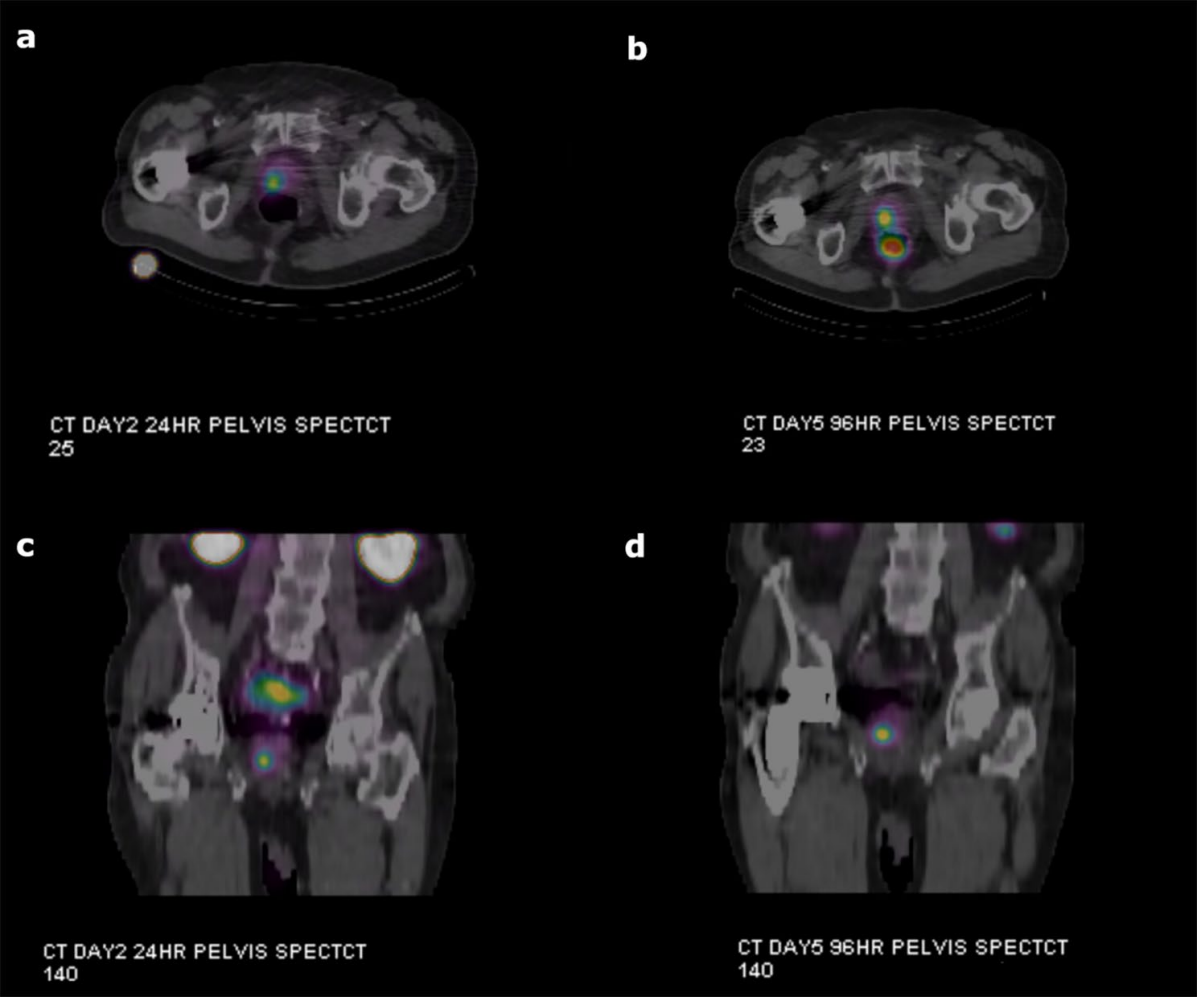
Post Lu-177 PSMA RLT SPECT/CT images Figures 3a and 3b show the axial views of the SPECT/CT images at 24 and 96 h post Lu-177 PSMA RLT administration. Figures 3c and 3d show the coronal views of the SPECT/CT images at 24 and 96 h post Lu-177 PSMA RLT administration. There was no uptake in the prostate on the SPECT/CT images taken 4 h after the Lu-177 PSMA RLT administration
